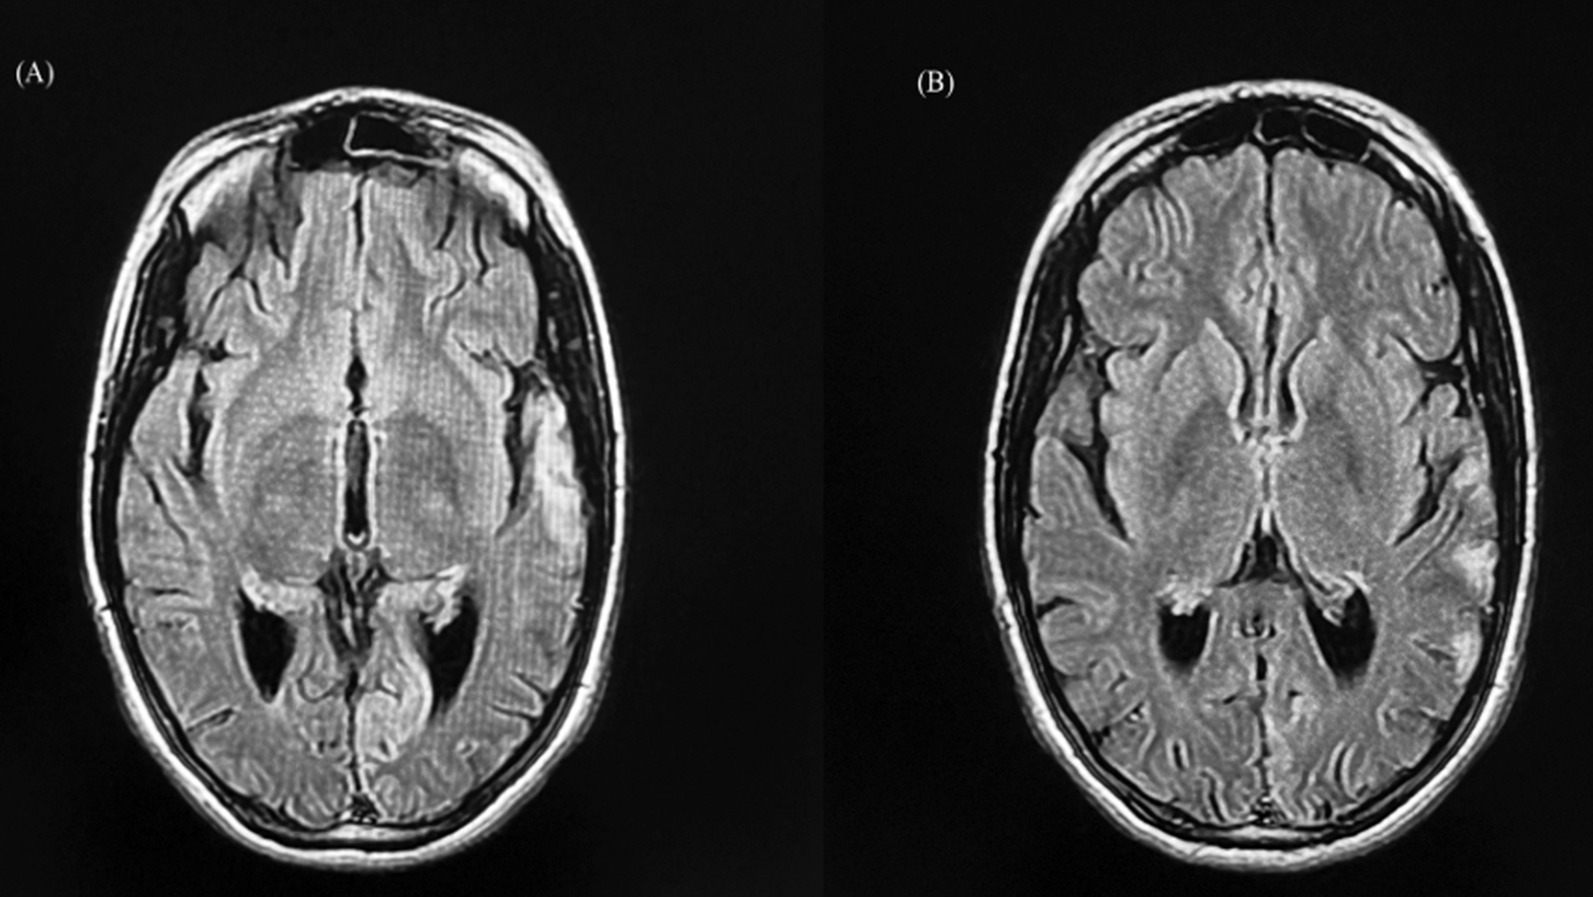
Brain MRI: (A) left temporal, parietal cortical and subcortical patchy areas of high signal intensity on T2 and Fluid attenuated inversion recovery Wigs (FLAIR WIGs). (B) left occipital and temporal patchy areas of high signal intensity
